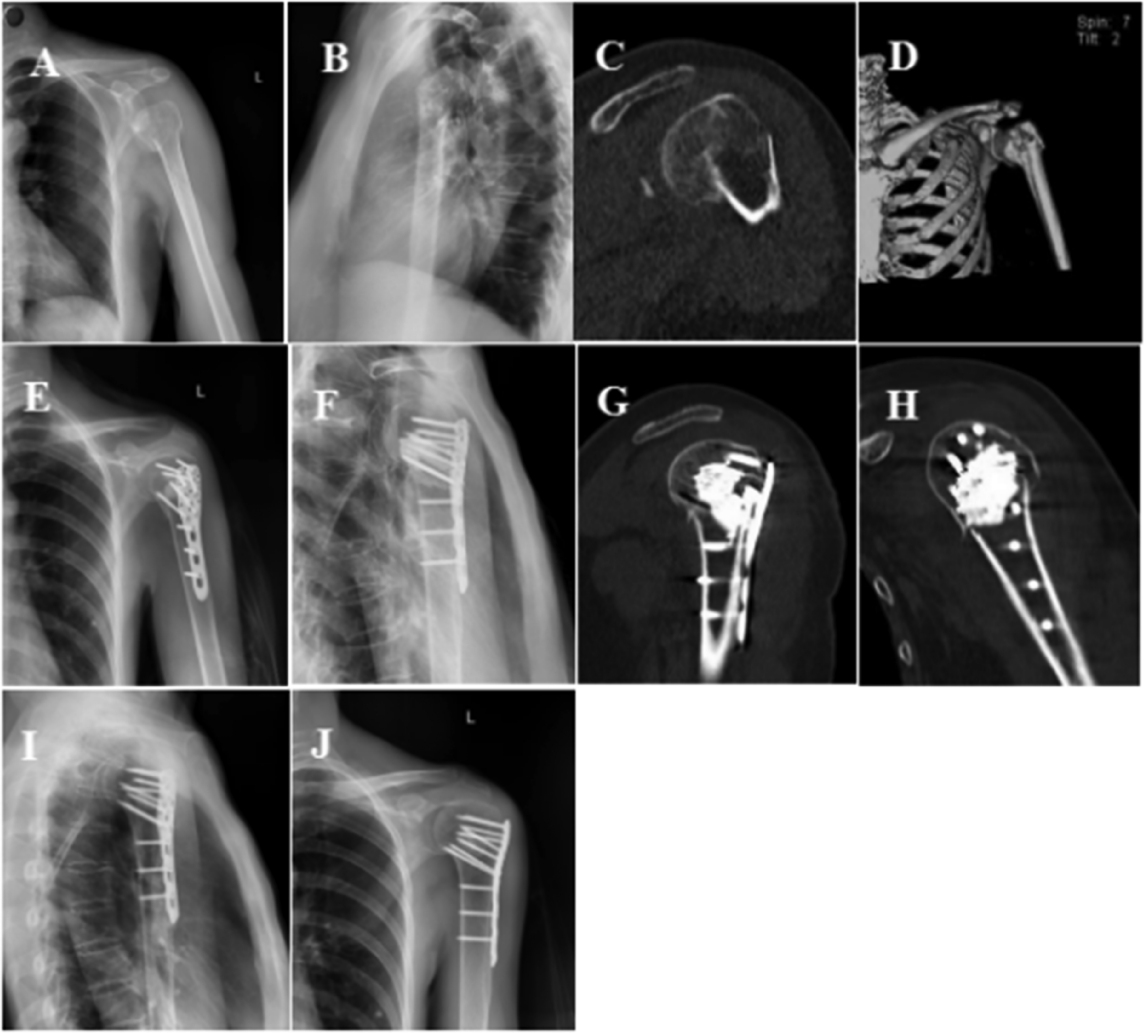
Follow-up imaging of comminuted fracture of proximal left humerus. (A,B) Preoperative X-rays showed fracture displacement, humeral head inversion, and displacement of large and small tubercles. (C,D) Preoperative CT scan of the left shoulder showed fracture inversion, separation and displacement of large and small nodules. (E,F) Postoperative x-rays showed that the fracture was well reduced and the humerus head was adequately filled with calcium sulfate injection. (G,H) Postoperative shoulder joint CT showed that injection of calcium sulfate filled the humerus skull defect adequately. (I,J) At the last follow-up, the fracture healed and calcium sulfate was completely degraded and absorbed.
